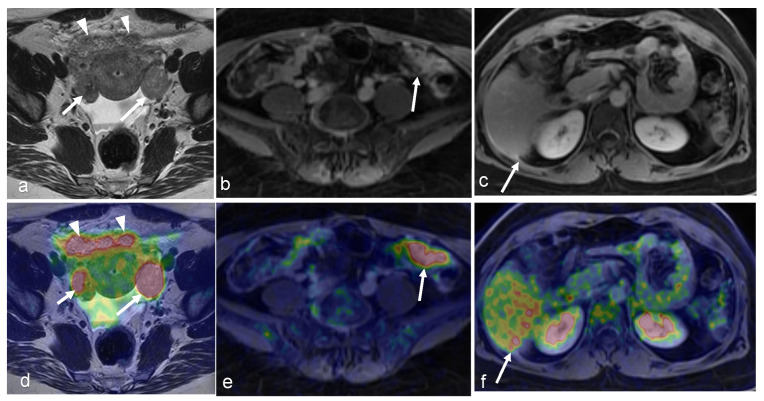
A 43-year-old woman with peritoneal carcinomatosis secondary to high-grade serous carcinoma has a surgical PCI score of 22. On an axial T2-weighted image, the bilateral ovarian tumors appear moderately hypointense (arrows in (a)) and show uptake of FDG on fused PET/MRI (arrows in (d)). Large omental caking ventral to the uterus and ovaries is also visible (arrowheads on (a,d)). Spread of the peritoneal implant to the left the paracolic gutter (in the lower abdomen (arrows in (b,e)) and the medial border of the right liver lobe can be observed (arrows in (c,f)) (Courtesy of Elisabeth Hedlund, MD, Håkan Ahlström, MD, and Björg Jónsdóttir, MD, PhD, Uppsala University, Uppsala, Sweden).
